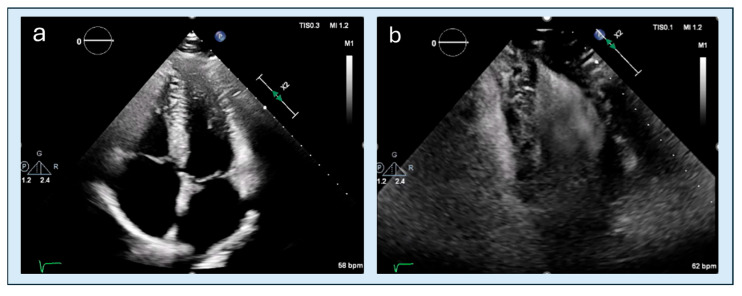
(a) Apical four-chamber echocardiographic view without the use of an intracavitary contrast agent. (b) Apical four-chamber echocardiographic view with the use of an intracavitary contrast agent. Contrast enhancement markedly improves endocardial border delineation and reveals the characteristic “spade-like” apical configuration, which is poorly defined on unenhanced imaging.
